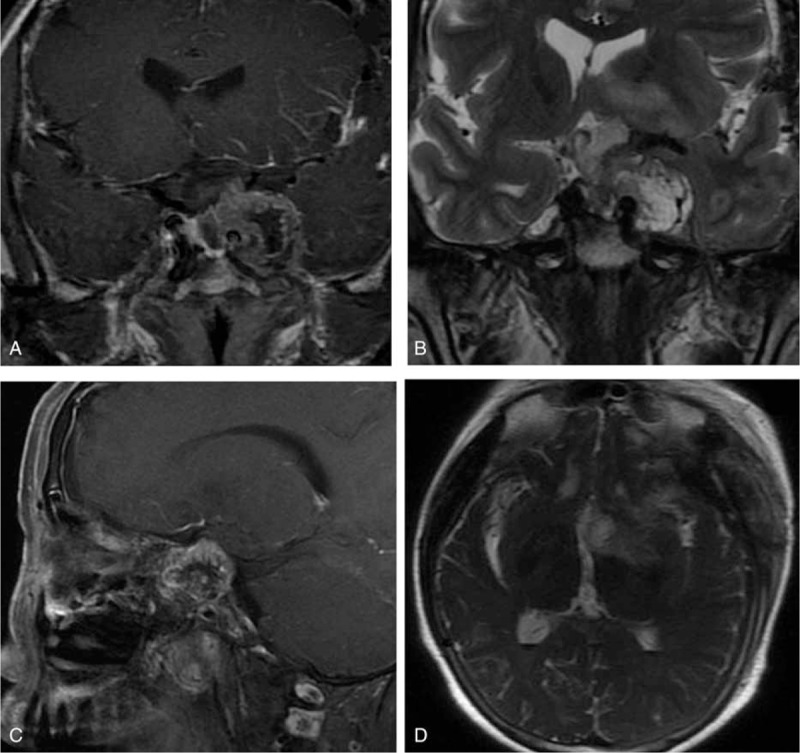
Postoperative MRI of the sellar region showed subtotal resection of the schwannoma. (A) coronal-enhanced T1WI; (B) coronal T2WI; (C) sagittal enhanced-T1WI; (D) axial T2WI.
